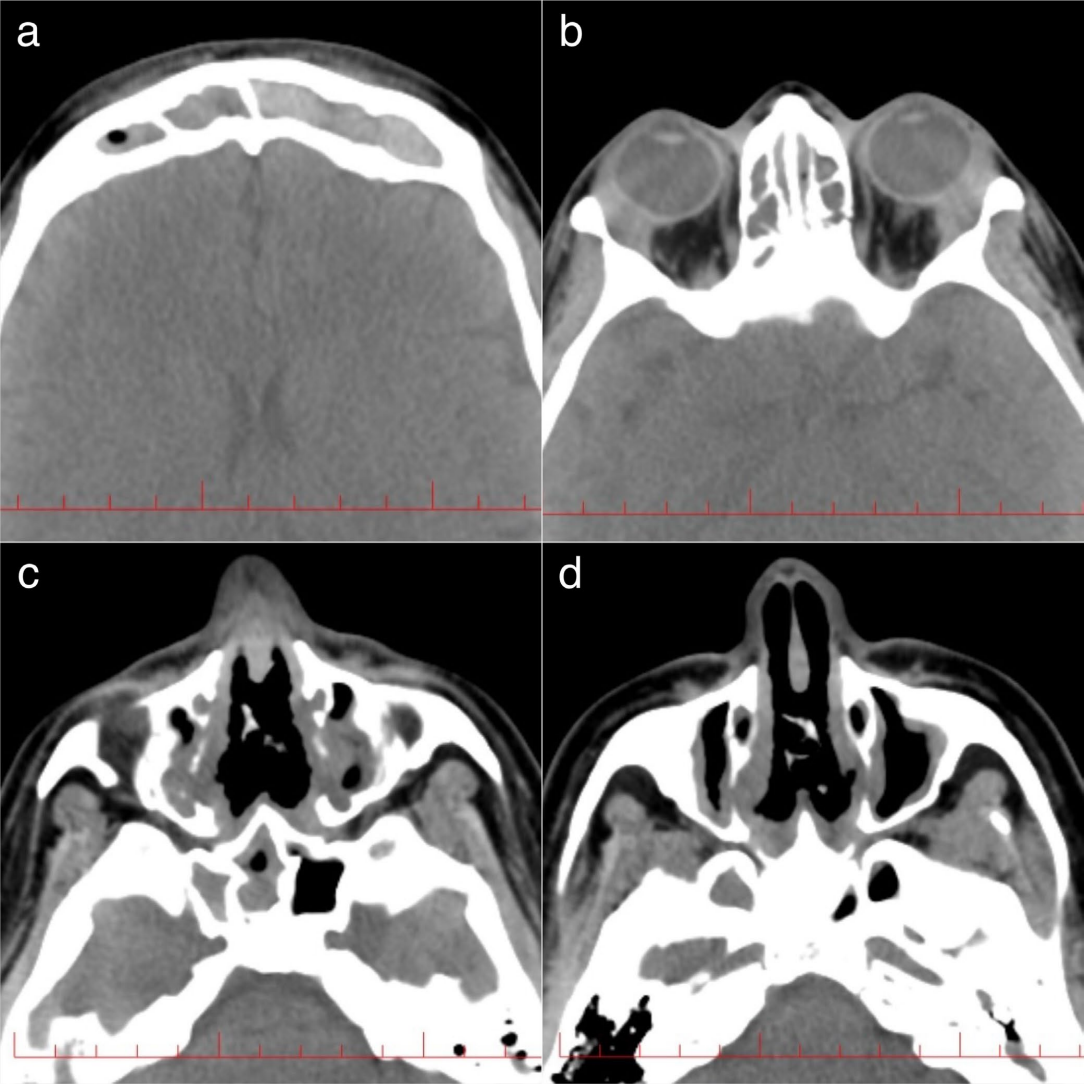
CT scan of paranasal sinuses indicating pansinusitis and nasal septum defect. (a) Frontal sinuses; (b) Ethmoidal sinuses; (c) Sphenoid and maxillary sinuses; (d) Nasal septum defect.
